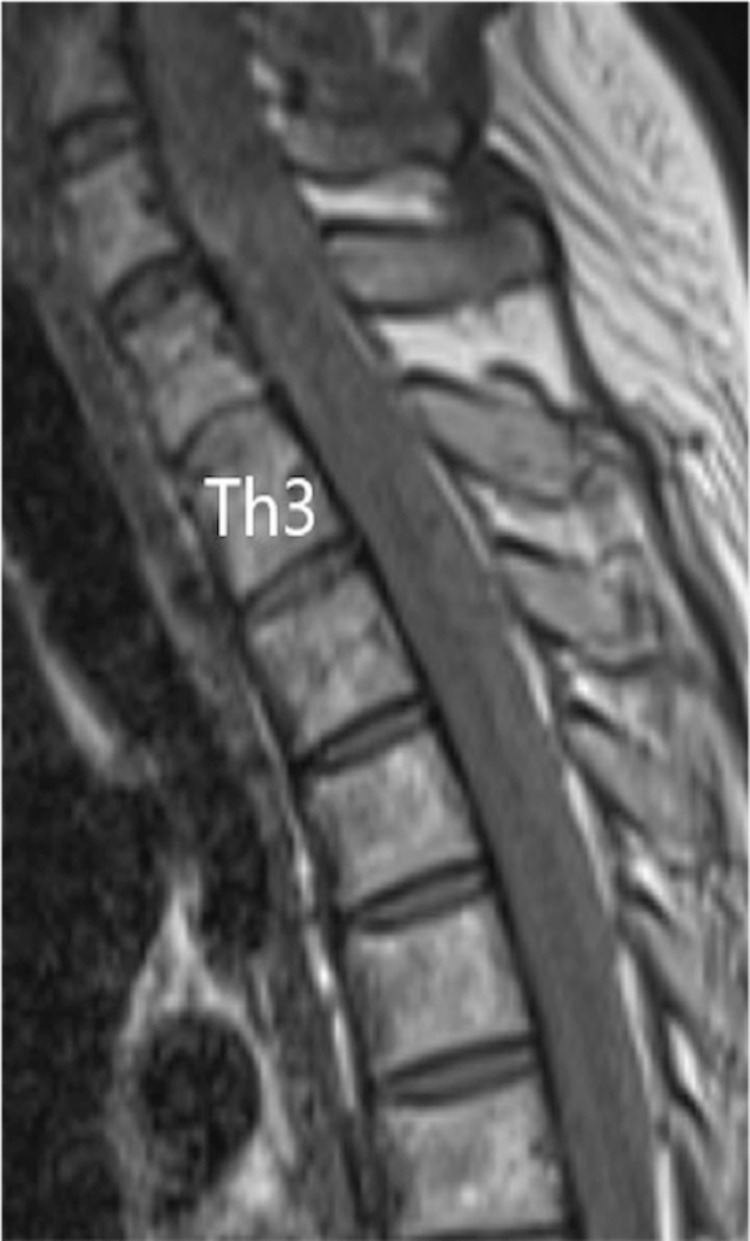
Sagittal T1-weighted MR images at the time of transfer A zonal change with a septum was observed on the right dorsal side of the spinal cord dura mater at the position from Th1 to Th6 whose T1-weighted image exhibited the signal equal to that of the spinal cord and higher than that of the cerebrospinal fluid.
